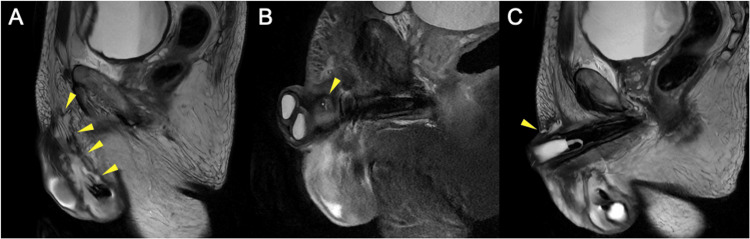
Sagittal MRI of the Penis Prior to Inflatable Penile Prosthesis Explantation (A) T2-weighted. High-signal fluid collection surrounding the prosthesis within the scrotum, extending superiorly towards the corpus cavernosum (arrowheads). (B) STIR. Small fistulous tract from the right corpus cavernosum (arrowhead), in continuity with the infected fluid collection (not shown). Induration of the scrotal and pubic soft tissues. (C) T2-weighted. Fistulous tract extending to the skin (arrowhead).
